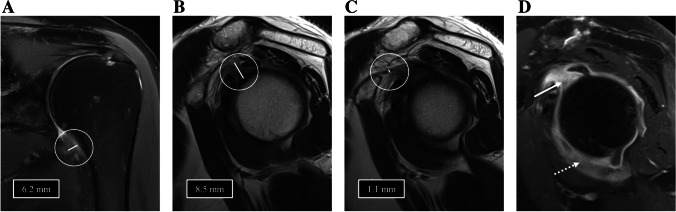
Images of the left shoulder of a 49-year-old male with clinical signs of ACS. A Coronal PD-weighted image shows a thickened capsule at the axillary recess (6.2 mm) with increased signal intensity while B T2-weighted image shows an increased thickness of the capsule at the rotatory interval (8.5 mm) indicating the presence of ACS. In contrast, the CHL is not thickened (1.1 mm), and the subcoracoid fat is not obliterated in T2-weighted sagittal imaging (C). D In T1-weighted fat-saturated contrast-enhanced sagittal image, axillary recess (dotted arrow) and rotatory interval (arrow) present with distinct contrast uptake confirming the diagnosis of ACS
